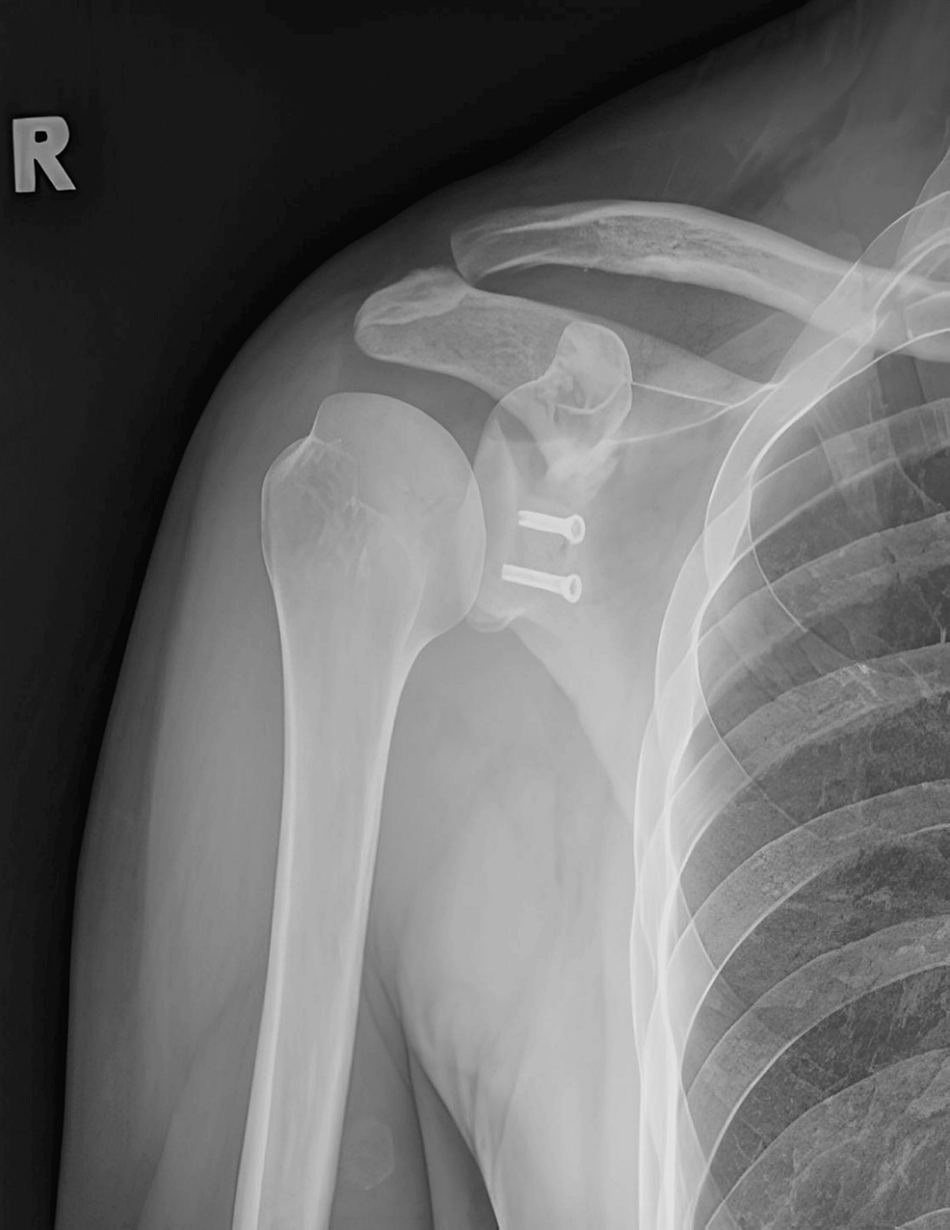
Radiograph (anterioposterior view) showing postoperative right shoulder Latarjet procedure.
